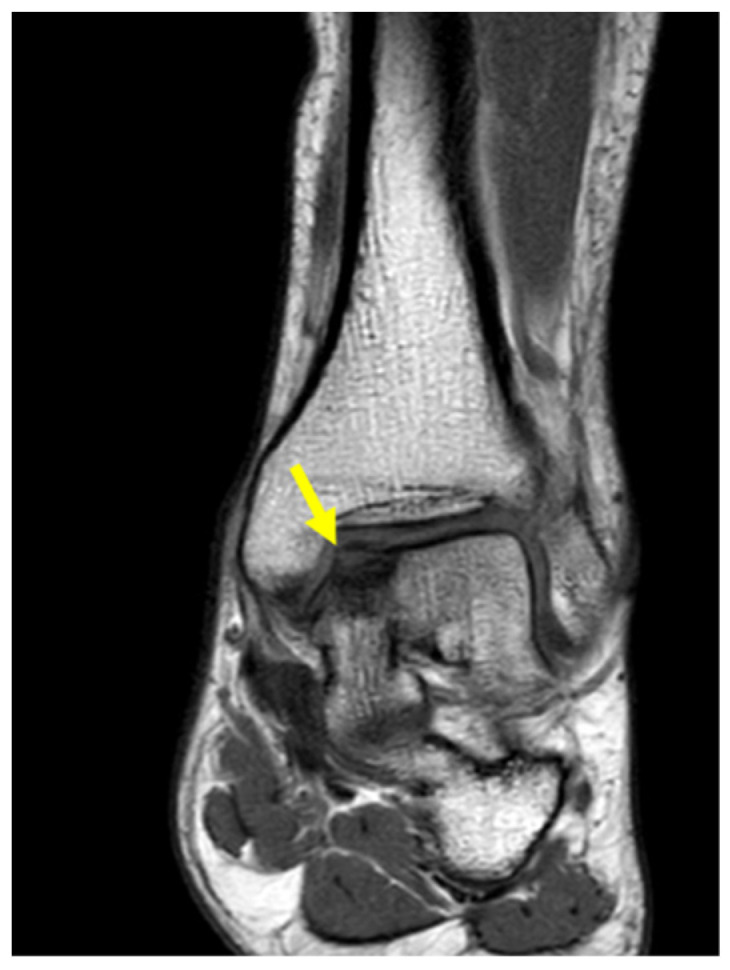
Coronal ankle MRI showing OCD (arrow) on the medial side of the left talus.
